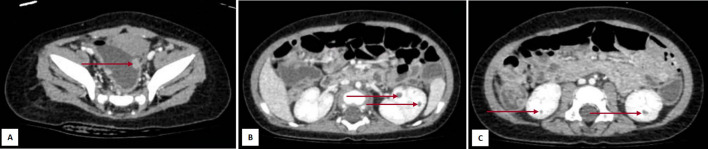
Images of the abdomen CT scan at diagnosis. (A) Polyp in the sigmoid. (B, C) Nodules in both kidneys. The red arrow in Panel (A) points to a polyp in the sigmoid colon; the red arrows in Panels (B, C) point to nodules in both kidneys.
